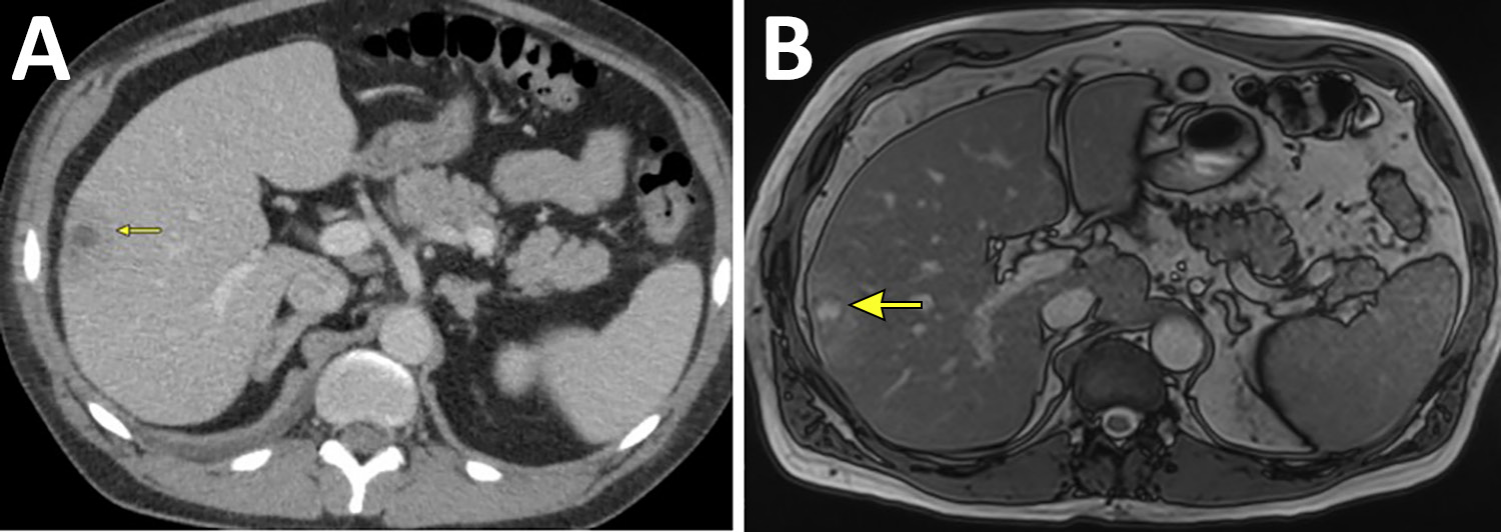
Imaging findings from a study of syphilis as a rare cause of pyogenic liver abscess in an HIV-positive man in Minnesota, USA. Computed tomography (A) and magnetic resonance imaging (B) of the abdomen and pelvis show rim-enhancing lesion (yellow arrows) in segments 5 and 6 of the liver.
